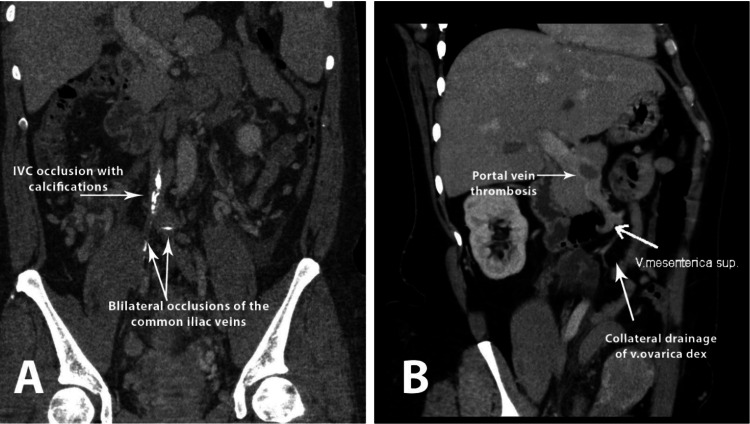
CT venography prior to the interventional treatment revealed chronic occlusion (A) IVC with calcifications and occlusion of the iliac and renal veins bilaterally. (B) Occlusion of the left ovarian vein and acute thrombosis of the portal vein and inferior mesenteric vein IVC: inferior vena cava
